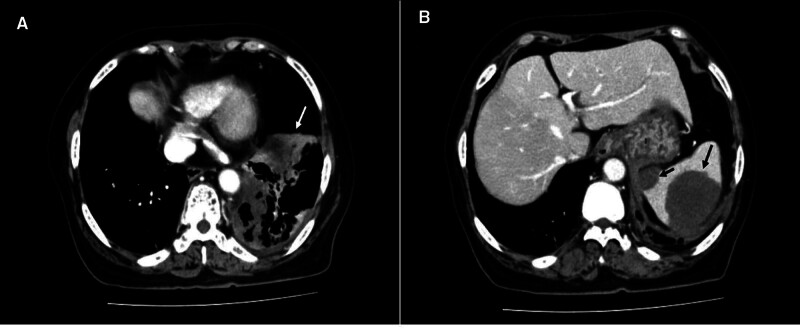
Enhanced CT of the abdomen. (A) Lung infection (white arrow). (B) Splenic abscess (black arrow). CT = computed tomography.
